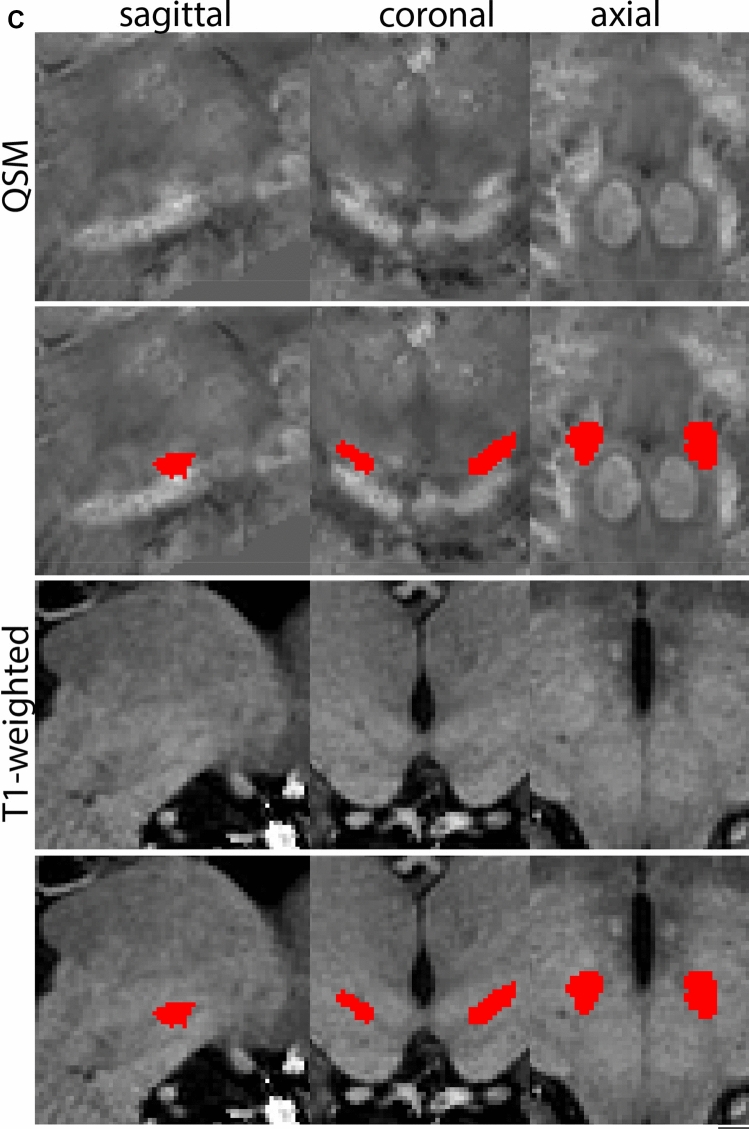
a The subthalamic nucleus (STN, red) appears as a hyperintense structure in the QSM contrasts due to its high-iron content. At these levels, the substantia nigra (SN) and red nucleus (RN) also appear hyperintense in the QSM contrasts, and can be used for anatomical orientation. Note the increased visibility of the STN/SN border in the coronal view. Additionally, note the limited visibility of these structures in the T1-weighted contrast. b More rostral view of the STN at the level of the mamillary bodies (visible in the T1-weighted contrast). Note the slightly more medial position of the STN at this coronal level. c Caudal level of the STN. Note the slightly more lateral position of the STN in the coronal view
